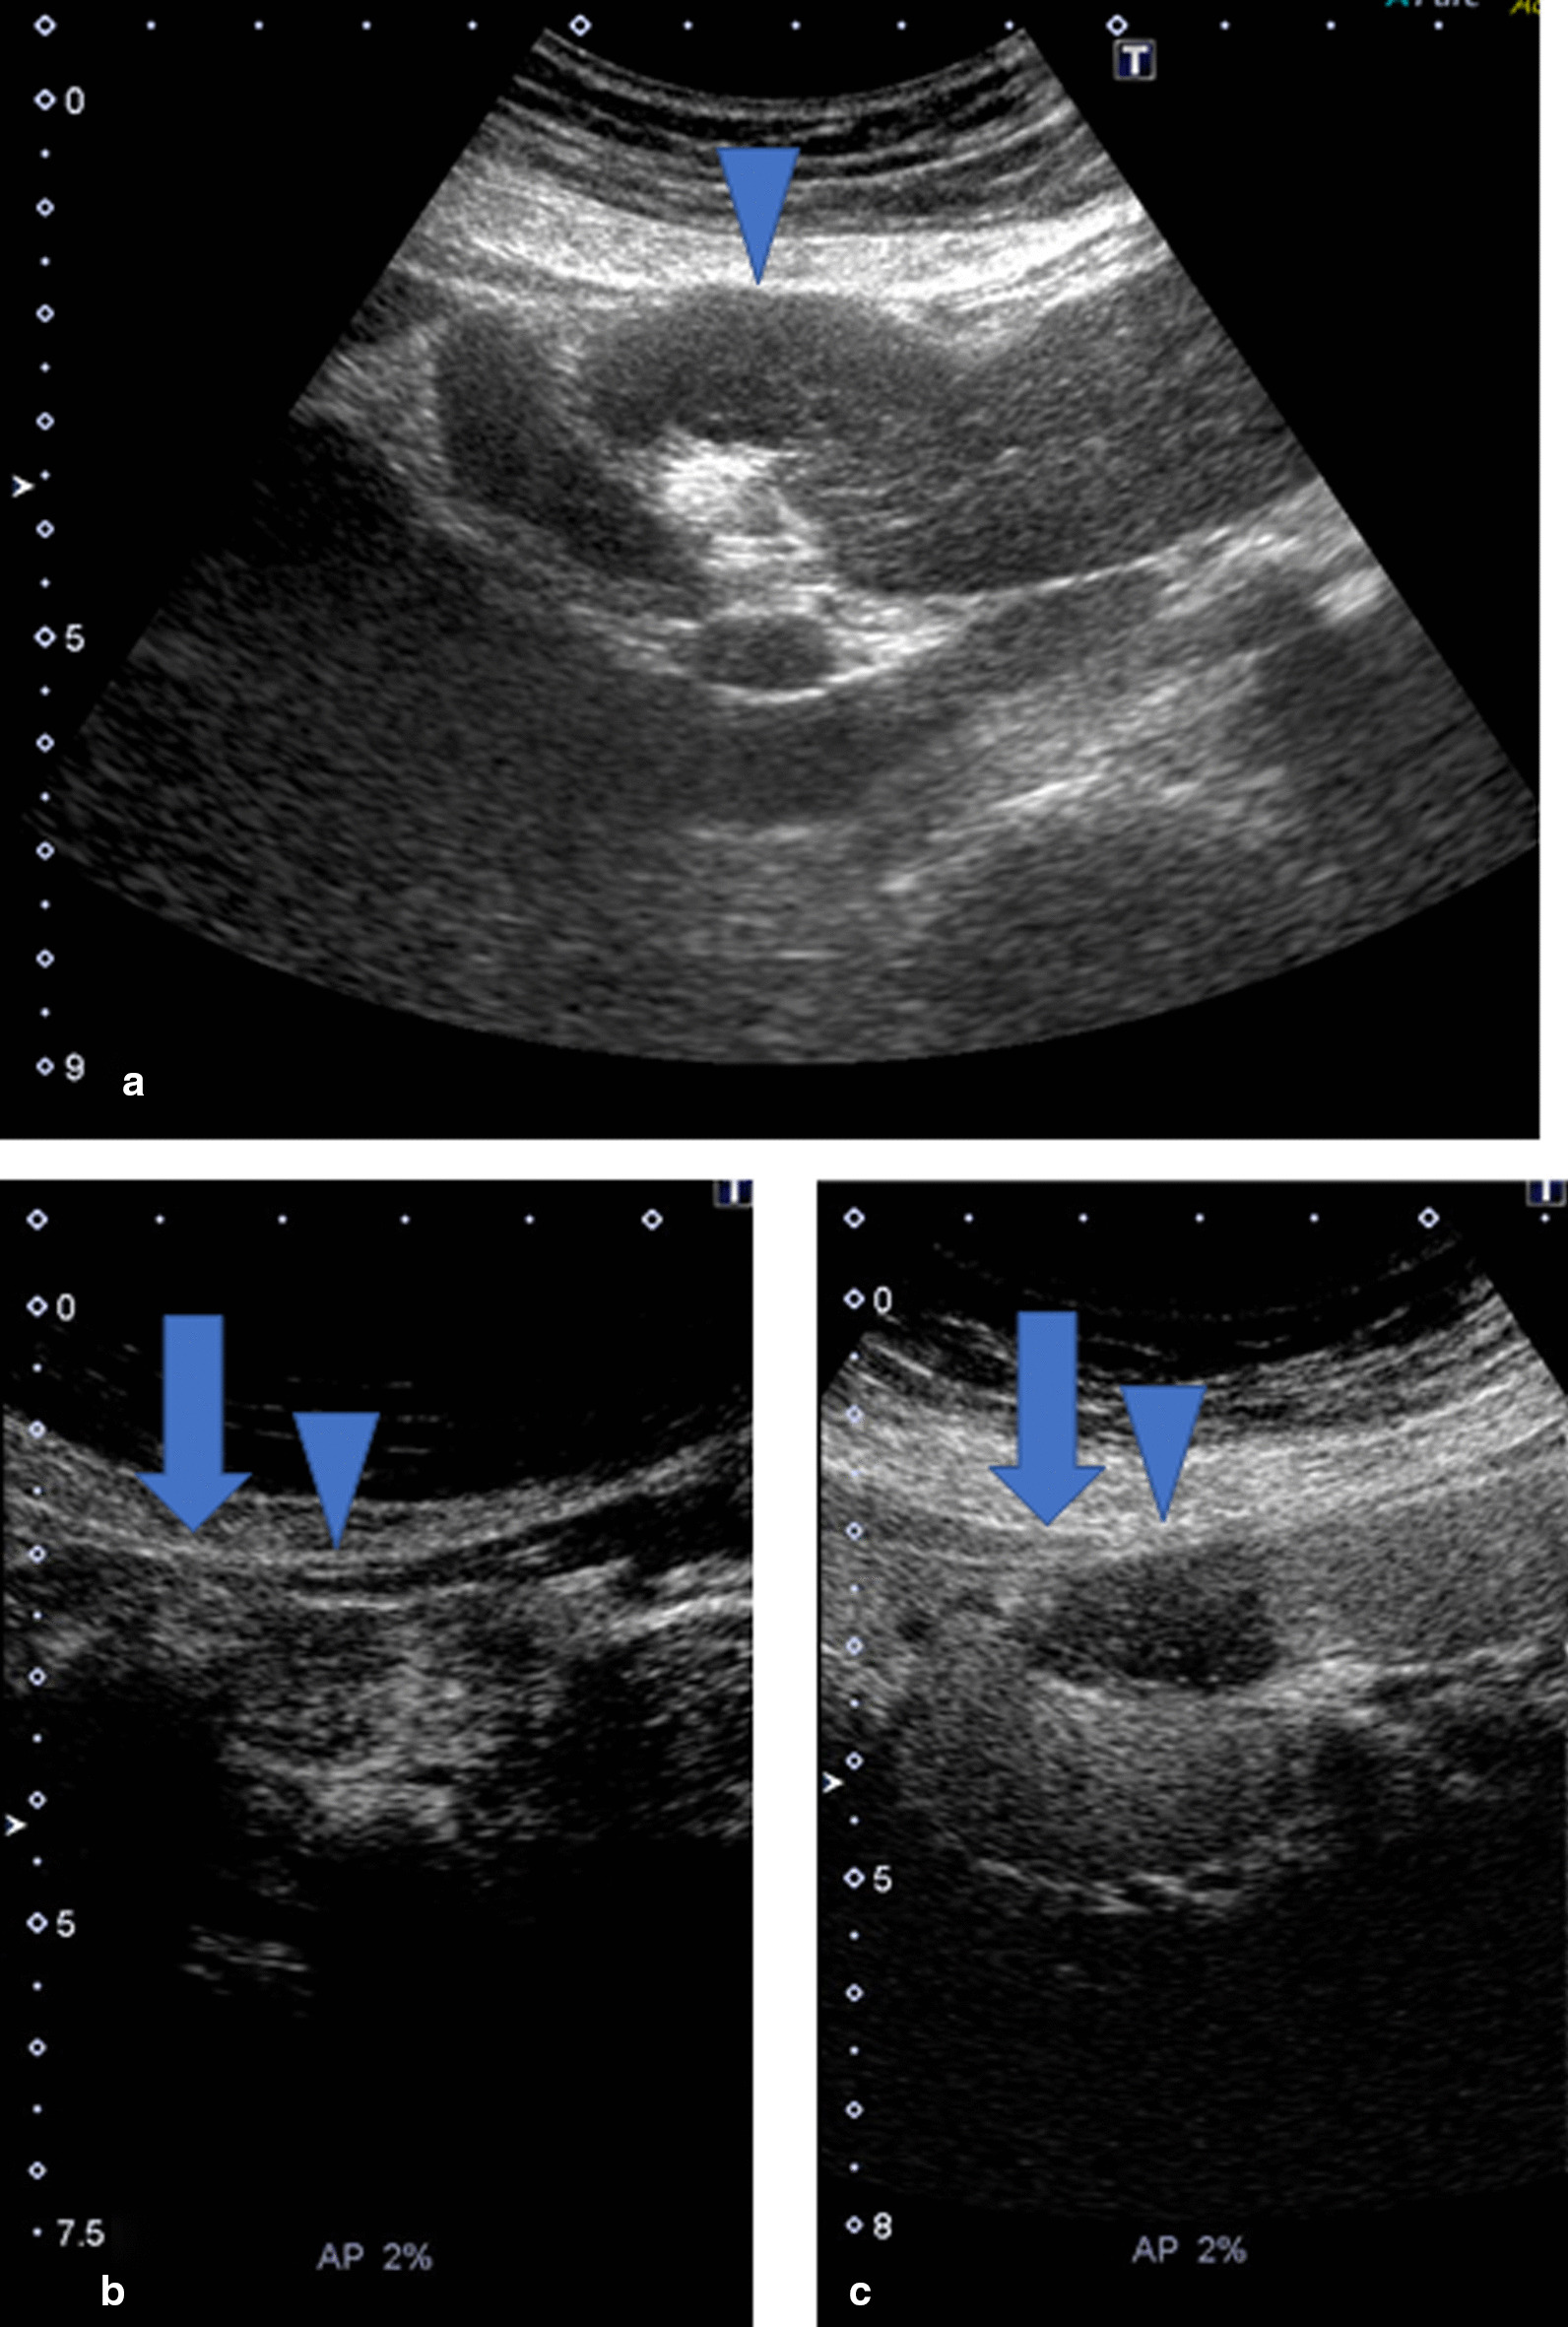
Ultrasound images of the tumor. a Ultrasound (US) examination of the protrusion area (arrowhead) in B-mode. b Contrast-enhanced US (CEUS), arterial phase. c CEUS, Kupffer phase. In CEUS, the tumor appears to have two components. The intrahepatic tumor (arrow, b and c) shows early enhancement and prolonged enhancement in the Kupffer phase. In the extrahepatic component (arrowhead, b and c), only the tumor margin is enhanced. The inside of the tumor shows spotty enhancement in the early phase
